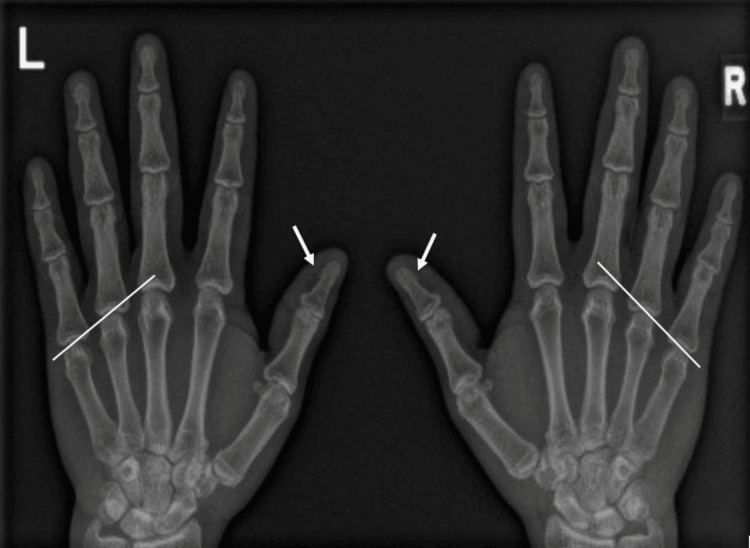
X-ray of both hands with no signs of brachydactyly, excluding AHO features. Normal hand X-ray demonstrating absence of skeletal abnormalities. A line drawn across the heads of the 4th and 5th metacarpals passes distal to the 3rd metacarpal head, indicating no metacarpal shortening. White arrows point to the distal phalanges of the thumb, showing normal bone length. AHO: Albright’s Hereditary Osteodystrophy
